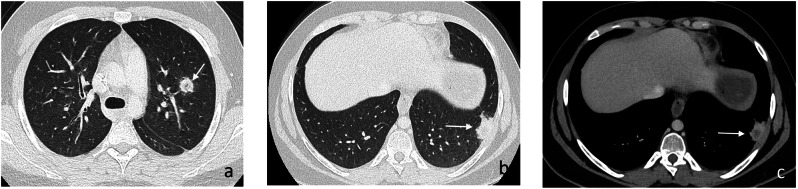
Proven pulmonary mucormycosis in a 17-year-old boy in agranulocytosis by acute myeloblastic leukemia (AML) under chemotherapy. Axial CE-CT images in lung (a, b) and mediastinal window (c) show a lung nodule with reversed halo sign in the left upper lobe LUL (arrows, a) and a subpleural located lesion in the posterior left lower lobe with “hypodense sign” (arrows, b, c) indicating central necrosis and probably the onset of cavitation. Note focal pleural infiltration
